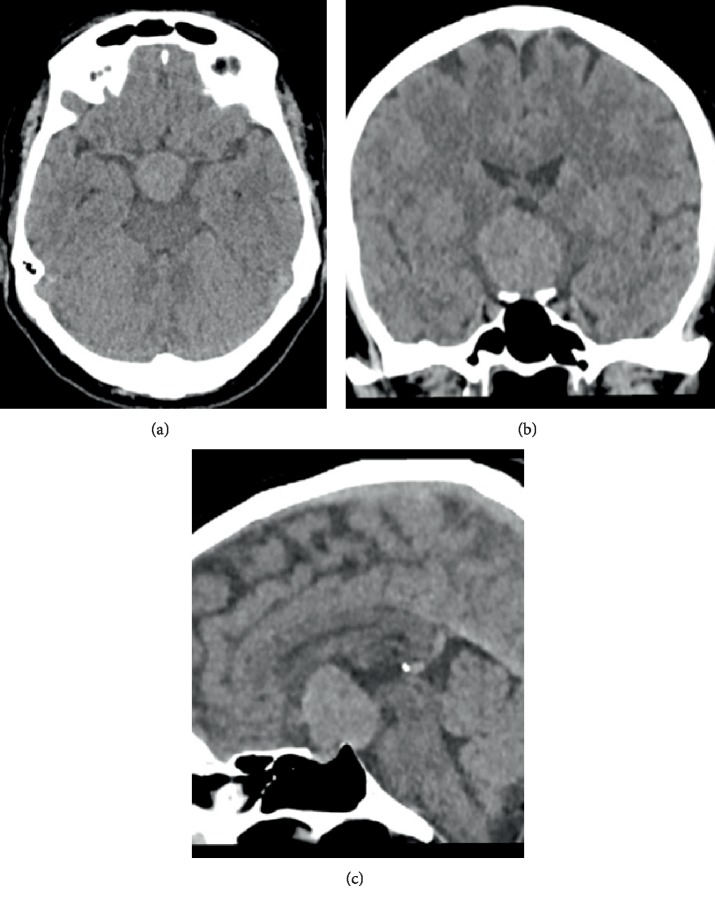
Initial contrast-enhanced brain CT. (a–c) Images show a voluminous well-circumscribed slightly hyperdense oval mass on the midline in the third ventricle, which has its largest diameter in craniocaudal direction.
